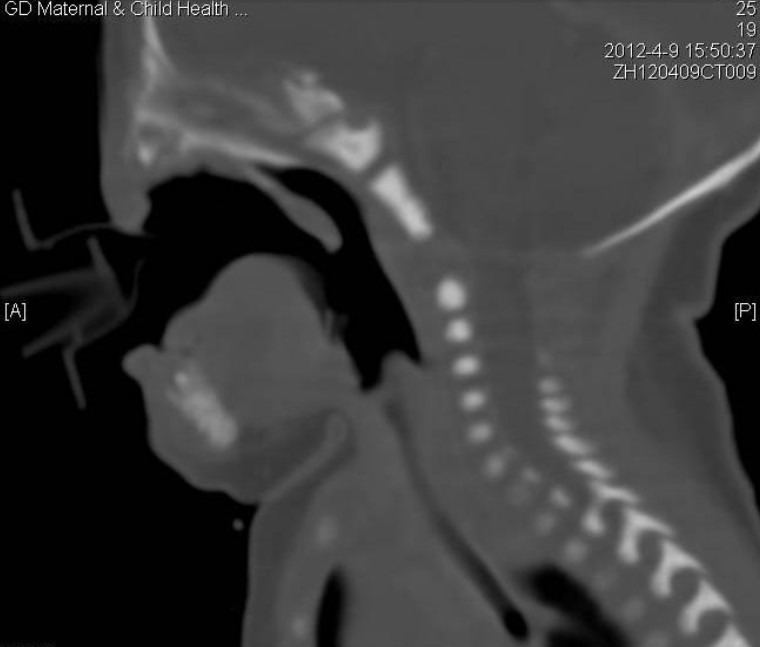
Figure 3: CT scan showing high arched palate, nostril atresia, the hypoplasia of bilateral maxillary sinus and ethmoid sinus
